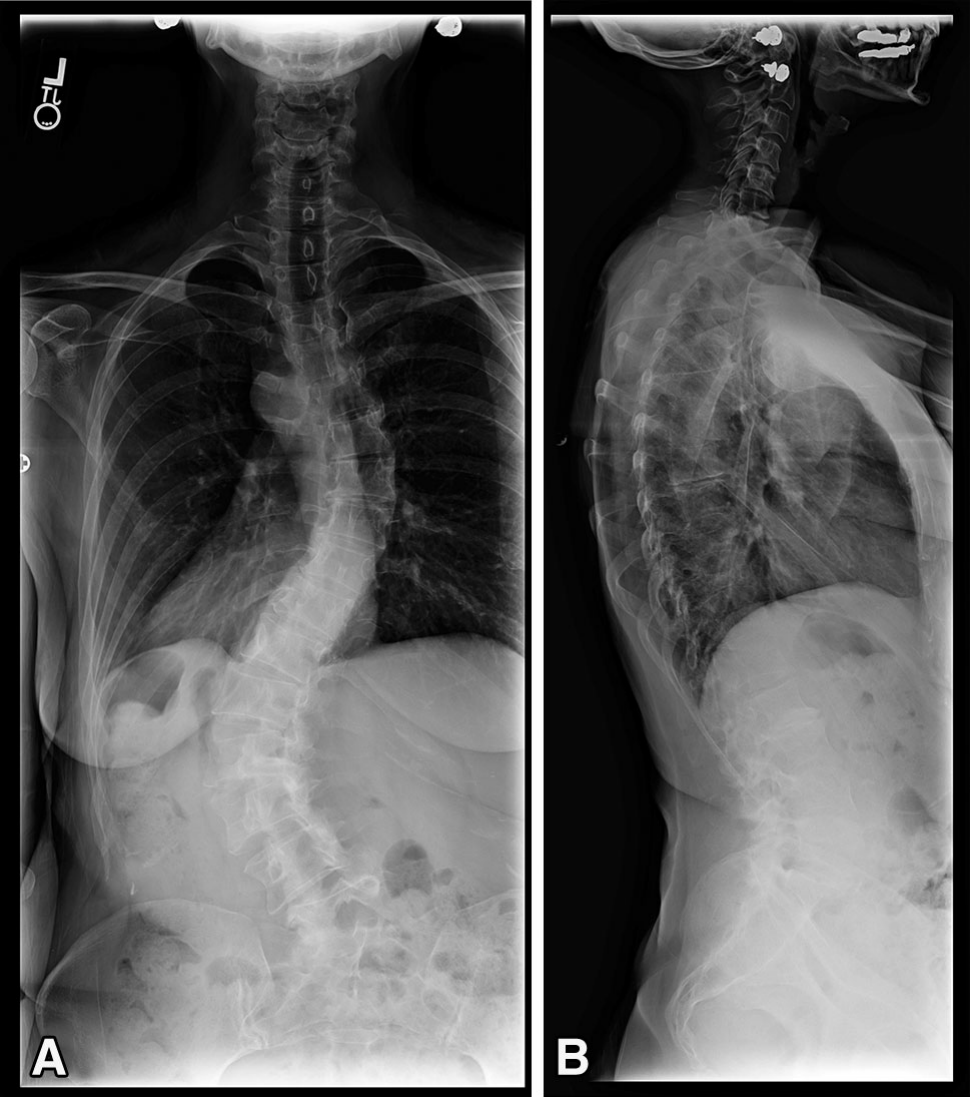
These 36-inch (A) AP and (B) lateral standing radiographs show the spine of a 53-year-old woman complaining of back and leg pain. Workup revealed her to have adult idiopathic scoliosis. She has a left curve from T10-L4 measuring 58°, a right curve from T5-T10 of 34°, and a fractional curve of L4-S1 measuring 33°.
